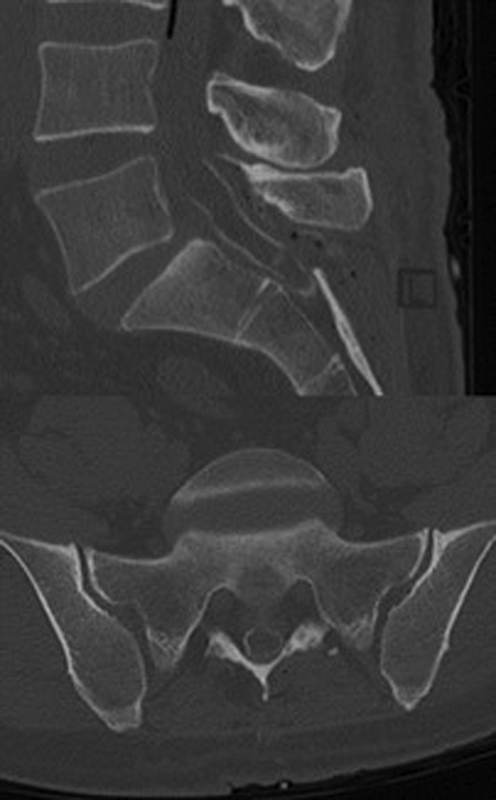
A bone window setting computed tomography (sagittal and axial view) performed postoperatively demonstrating the full extent of the remaining intradural bone formation.
